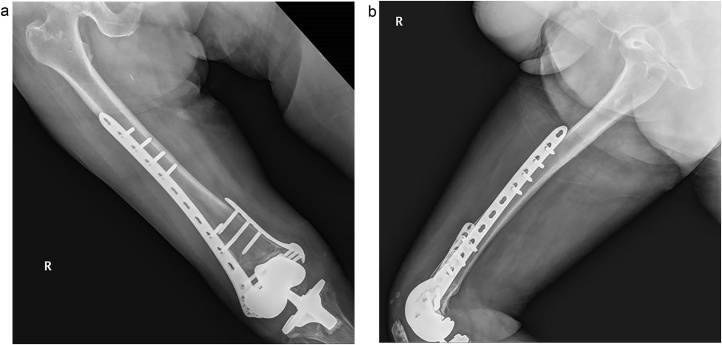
Radiographs at postoperative 6 months, showed a complete fracture healing with dual plating. (A) anterioposterior radiograph (B) lateral radiograph.
